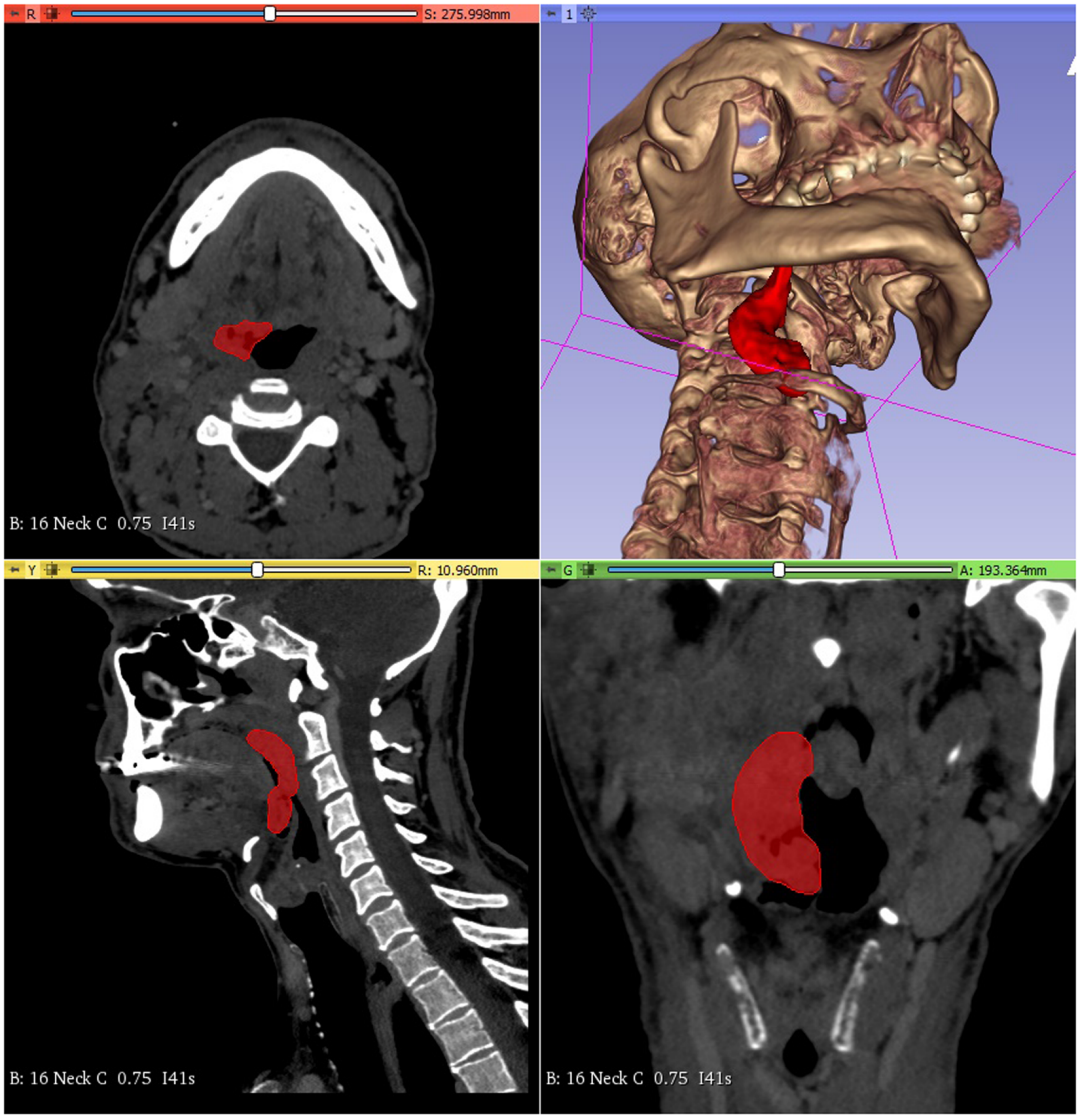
HR CT image and 3D model of a HPV negative stage T3 tonsillar squamous cell carcinoma. Tumor mass is marked red on the horizontal, sagittal and frontal planes in all sections and a 3D model is built up based on the CT segments on the upper right quarter of the picture.
